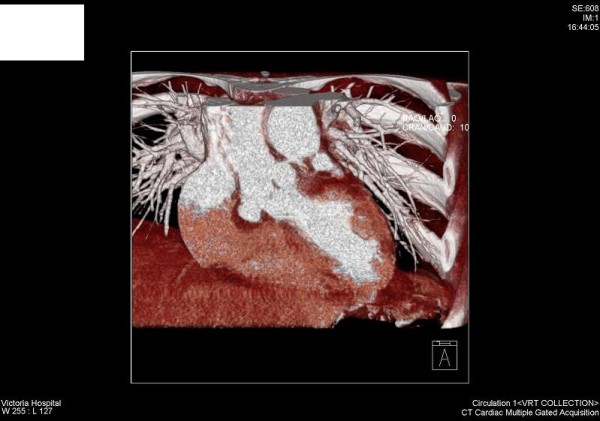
CT scan showing: non coronary cusp aneurysm with intramural thrombus.
